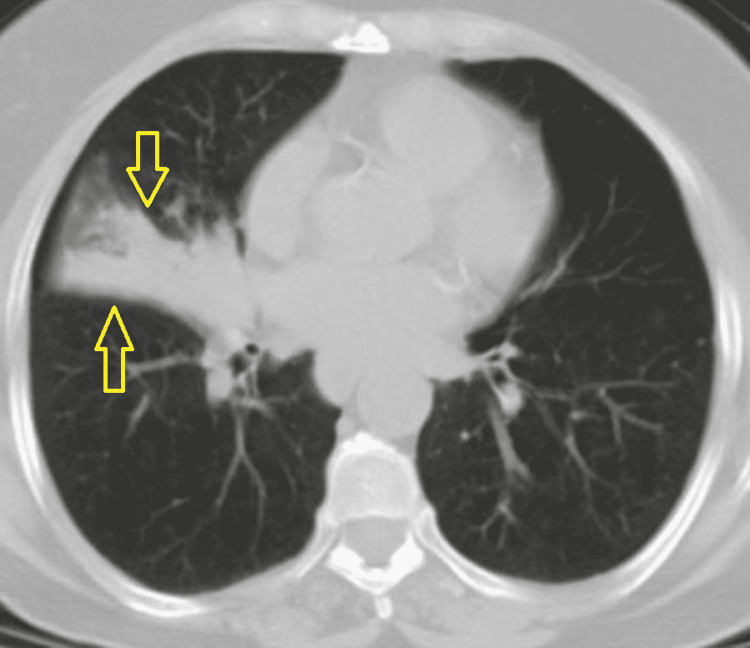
CT scan of the chest showing right middle lobe consolidation
